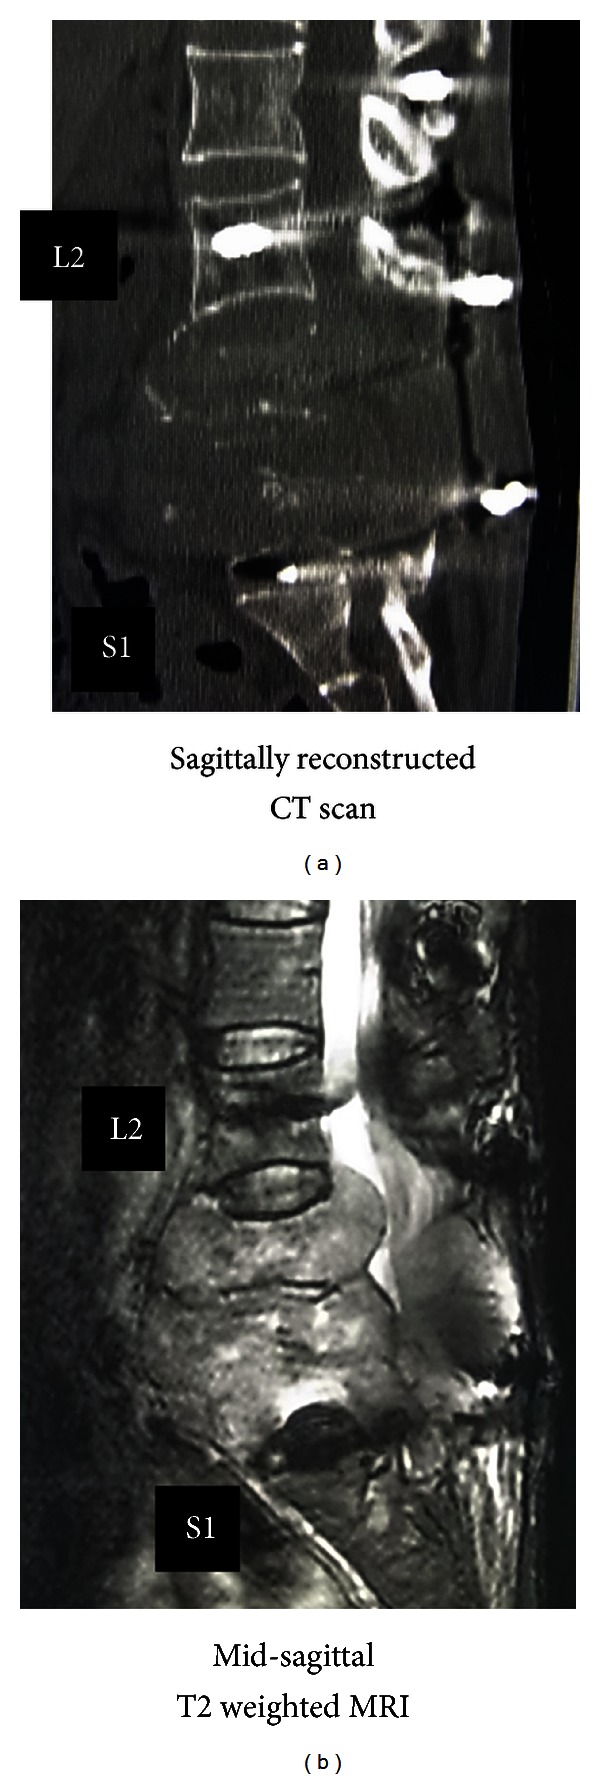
CT and MRI before the second surgery (February 2010), around 3 years after the first surgery. Sagittally reconstructed CT images (a) revealed complete destruction by the tumor of the L3, L4, and L5 vertebrae including the vertebral bodies and laminae.
